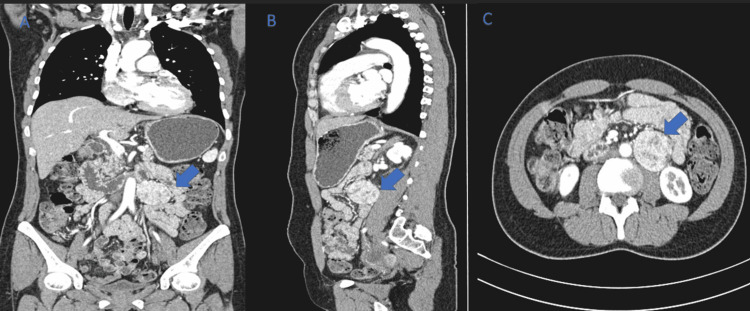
Thoraco-abdominopelvic CT scans. (A) Coronal section; (B) sagittal section, and (C) transverse section of the abdominal CT showing a well-defined heterogeneous hypodense mass in the left para-aortic region above the level aortic bifurcation enhanced after injection of the contrast product. A 42 mm x 41 mm lesion without signs of loco-regional invasion was measured.
